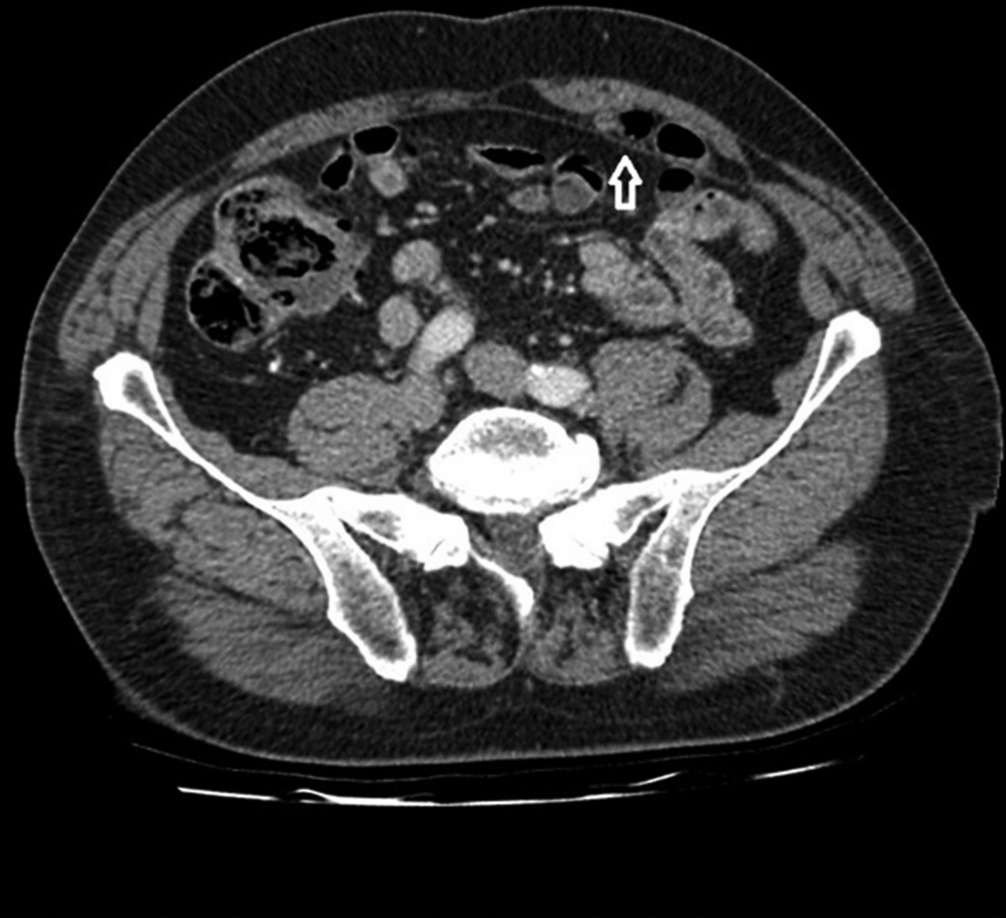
An additional contrast-enhanced, portal venous phase axial CT demonstrates the herniated bowel loops (arrow). The loops are not dilated and hence there is no bowel obstruction.
